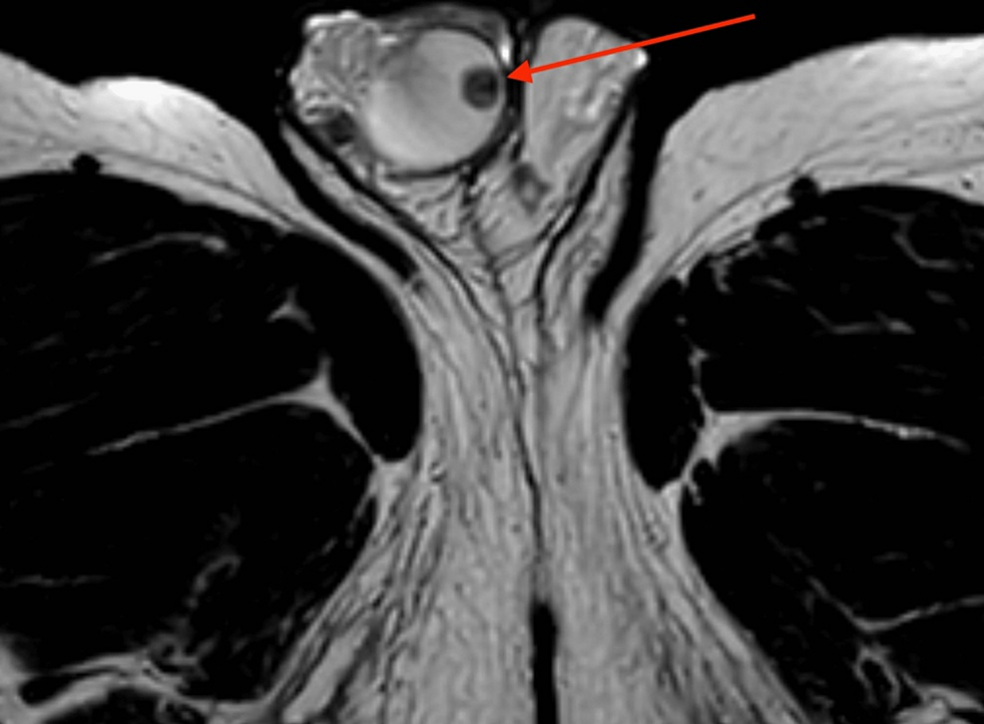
T2-weighted transversal MRI of the pelvis Image of the scrotum and right testicle and transversal view of both thighs showing an inhomogeneous, hypo-intense mass with a smooth border in the right testicle (marked with the arrow)
